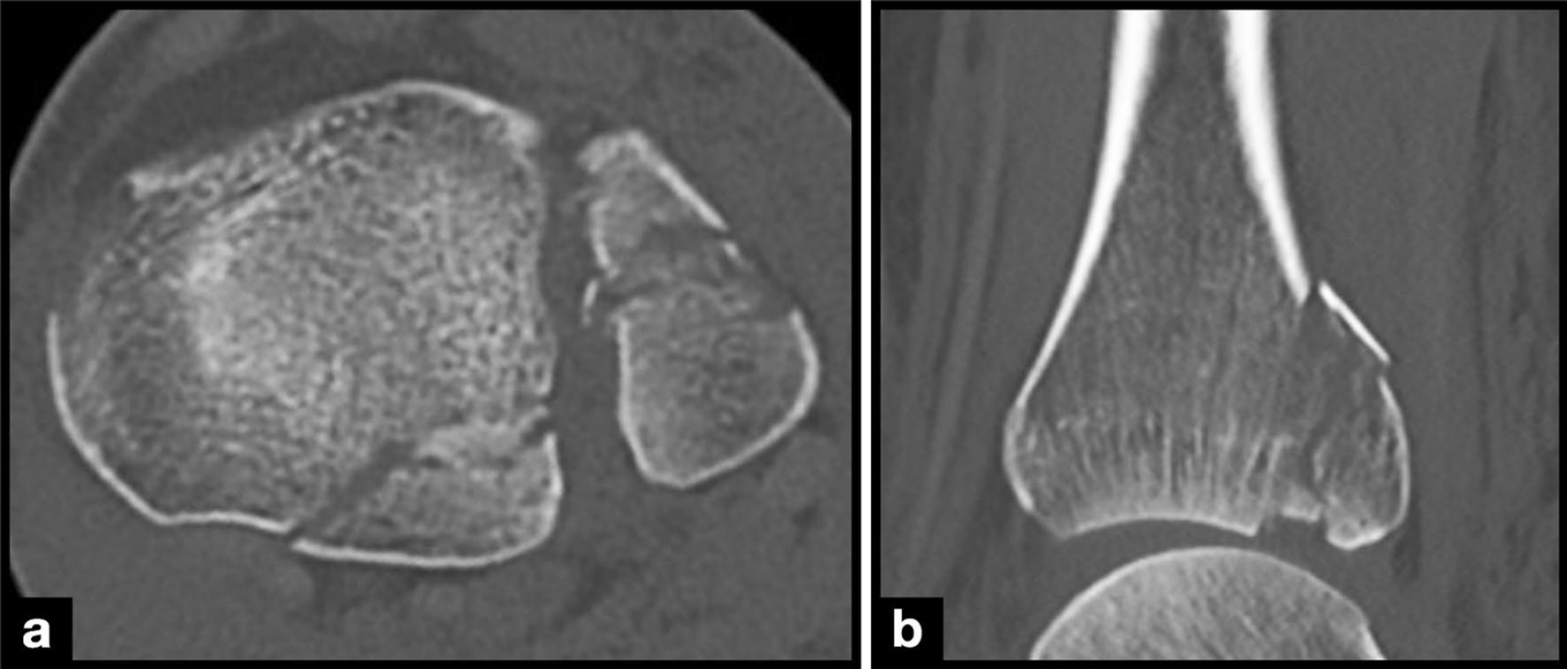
CT scan with a dislocated intercalary fragment in the a axial and b sagittal plane
